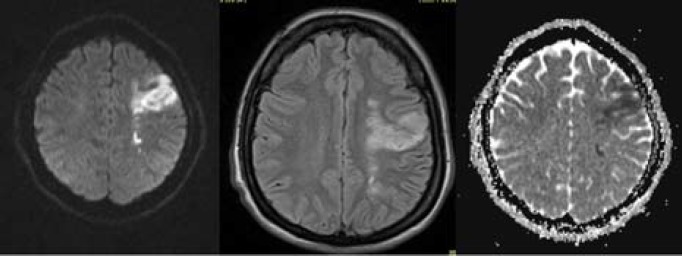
Left internal borderzone and middle cerebral artery (MCA) infarction in magnetic resonance imaging (MRI) study
